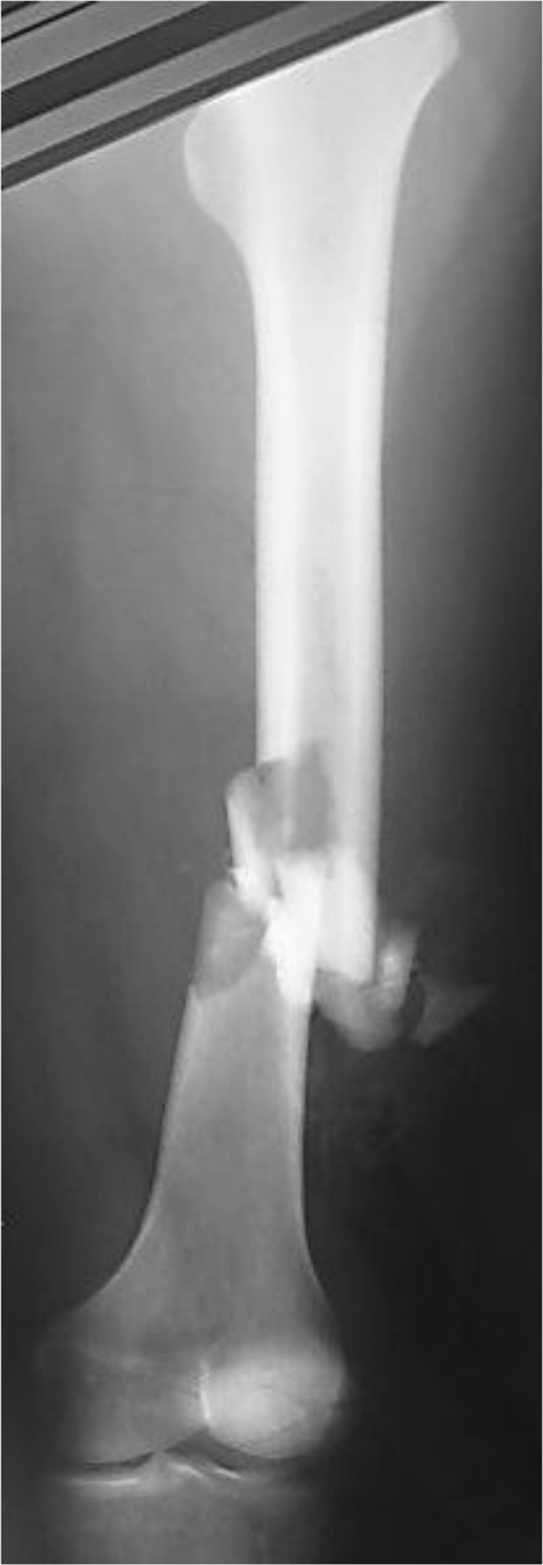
Preoperative radiographs. The femoral diaphyseal fracture (OTA 1, 32-C1.3).
